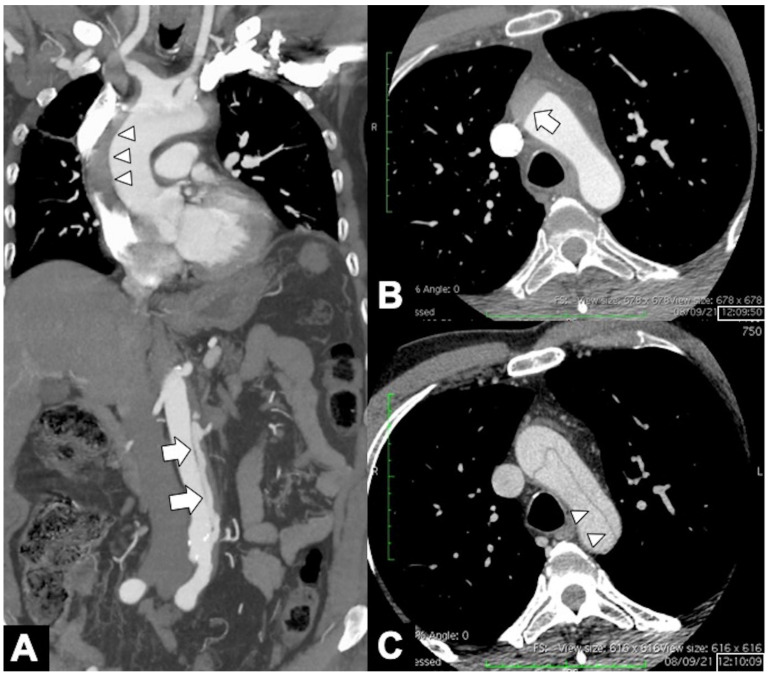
(A) Coronal MPR image of CTA in a 57-year-old man with chest pain and troponin negative shows coexistence of an acute intramural hematoma in the ascending aorta (type A IMH, arrowheads) and a dual-barrel dissection in the abdominal aorta (arrows). (B,C) Axial CTA images in another patient, showing the extension of the dissection flap from the proximal arch (arrow) to its distal segment (arrowheads) after only 19 s in the same CTA examination. Aortic dissection may be a very dynamic process.
